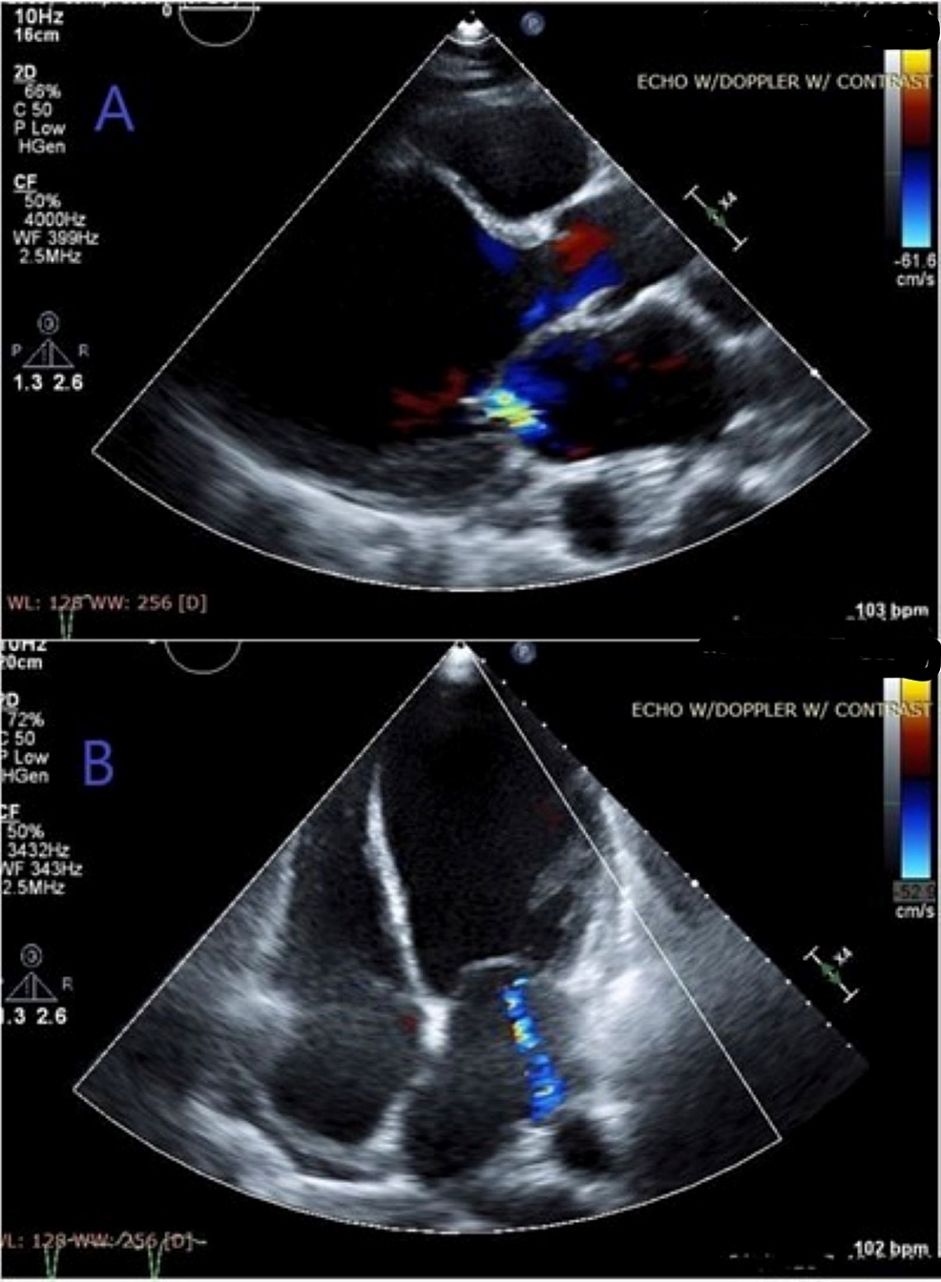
Transthoracic echocardiogram demonstrating mitral regurgitation (arrow) and dilated left ventricle in (A) parasternal long-axis view and (B) apical four-chamber view.
